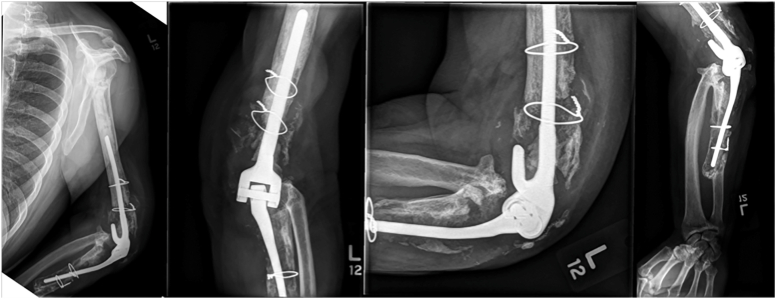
Pre-operative radiographs (2017) showing a ballooned proximal ulna with severe segmental bone loss, progressive humeral periprosthetic lucency, and a mature radioulnar synostosis.
